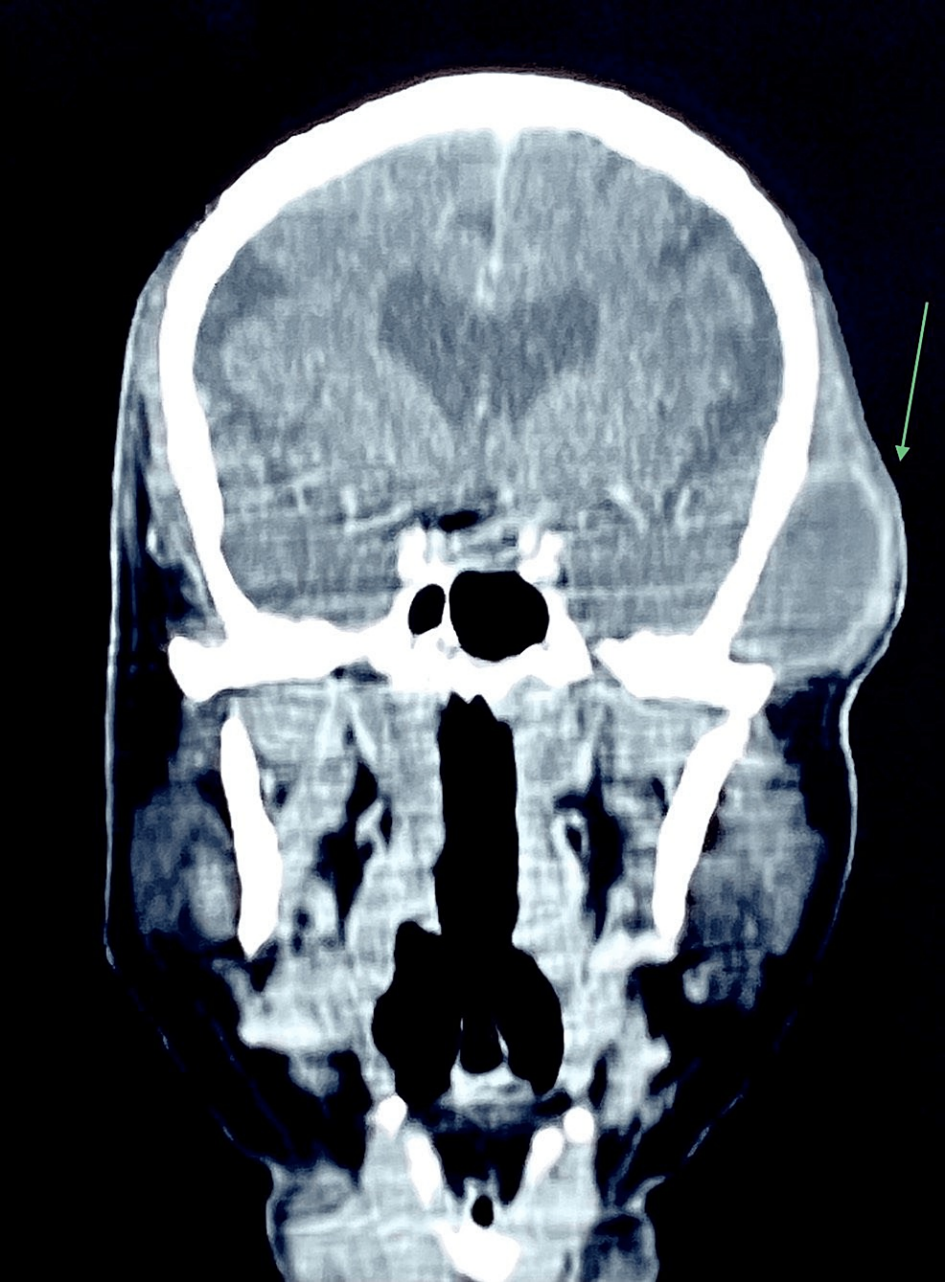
Section of Computed Tomography (CT) showing lesion in the left temporal region. The arrow in the coronal view of the CT shows a well-defined cystic lesion in the left temporal region.
